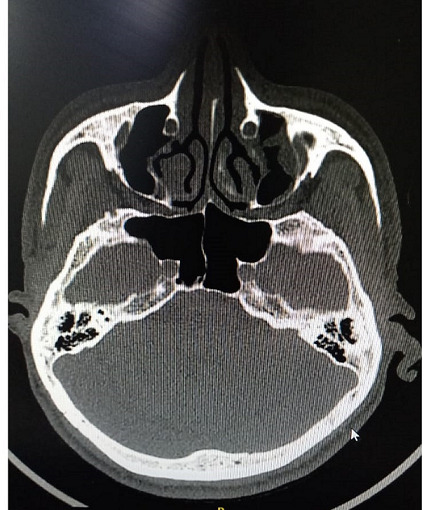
Case 2. CT PNS: mucosal thickening in the ethmoidal and maxillary nasal sinuses.
